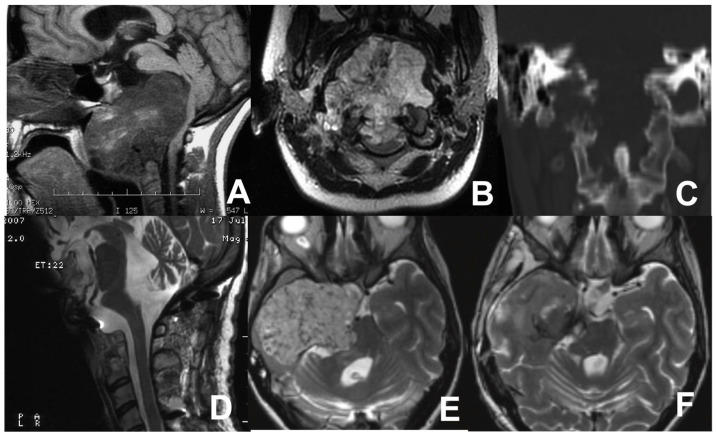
MRI sagittal (A) and coronal (B) views showing the huge chordoma in the lower third of the clivus, extending to the body of C2, causing posterior displacement of the brainstem, and compressing the nasopharynx. CT scan (C) showing osseous erosion of the occipital condyles, anterior arch of C1, and a portion of the C2 body. Postoperative MR scan after first operation (D). Pre- (E) and postoperative (F) axial MRI scans after the tumor relapse involving the clivus, sphenoid region, right petrous bone, and temporal fossa with severe compression of the brainstem.
